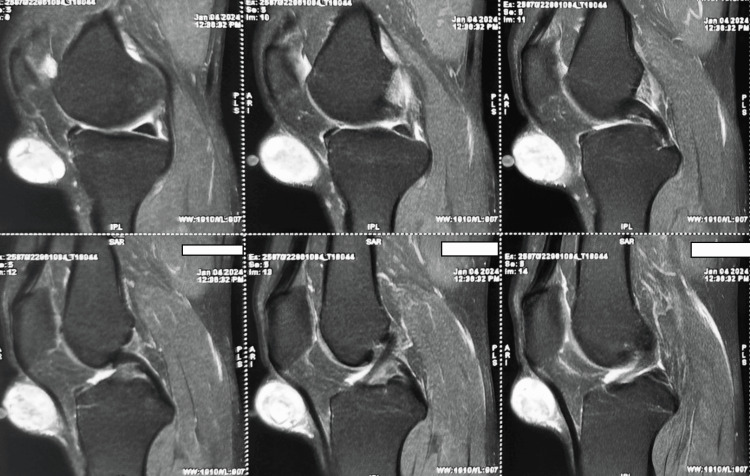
Sagittal T2- and T1-weighted MRI showing an infrapatellar sebaceous cyst MRI, magnetic resonance imaging
